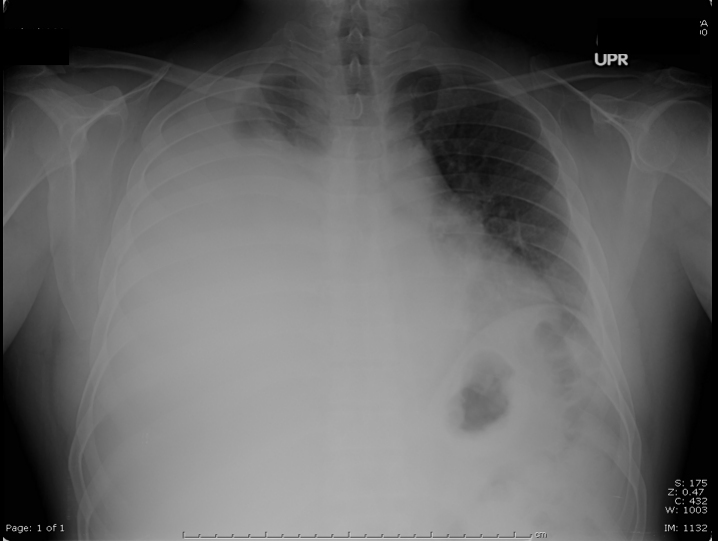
Chest radiograph showing moderate right pleural effusion.
